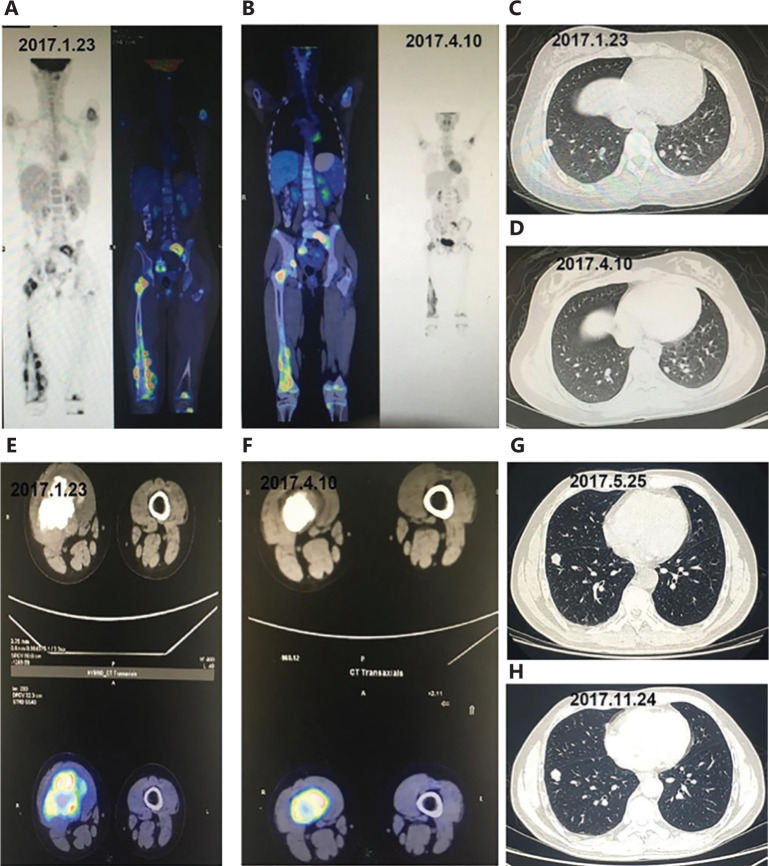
Comparison of imaging findings between 2 patients treated with apatinib for stage IV osteogenic sarcoma that did not respond to chemotherapy. (A-F) Efficacy in patients who were diagnosed with stage IV osteosarcoma at the first visit and received apatinib after they did not respond to first-line chemotherapy. (A, C, E) Imaging findings before treatment with apatinib (January 23, 2017). (B, D, F) Positron emission tomography-CT showed significant decreases in the sizes and metabolic activity of the tumors after treatment with apatinib (April 10, 2017). (G-H) Effects of apatinib in patients with metastatic Ewing’s sarcoma in the lungs who did not respond to first-line chemotherapy. (G) CT images of the chest before treatment with apatinib (May 25, 2017). (H) The lesions in the lungs remained stable from initiation of oral use of apatinib on May 25, 2017 to re-examination on November 24, 2017.
